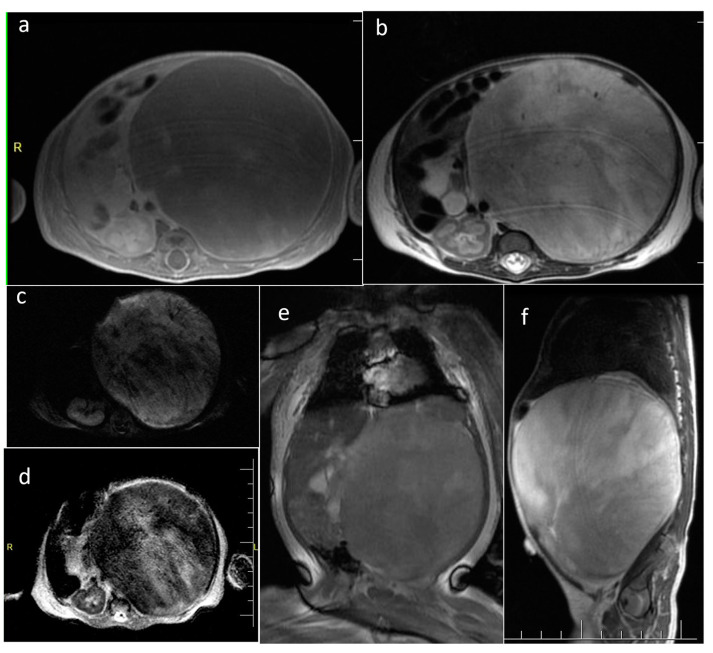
Wilms tumor arising from the left kidney in a newborn—encapsulated, heterogenous with necrosis, mass effect, contrast enhancement and restricted diffusion in solid parts. (a) Axial CE-T1WI; (b) axial T2WI; (c) DWI; (d) corresponding ADC map; (e) coronal FIESTA; (f) sagittal T2WI.
